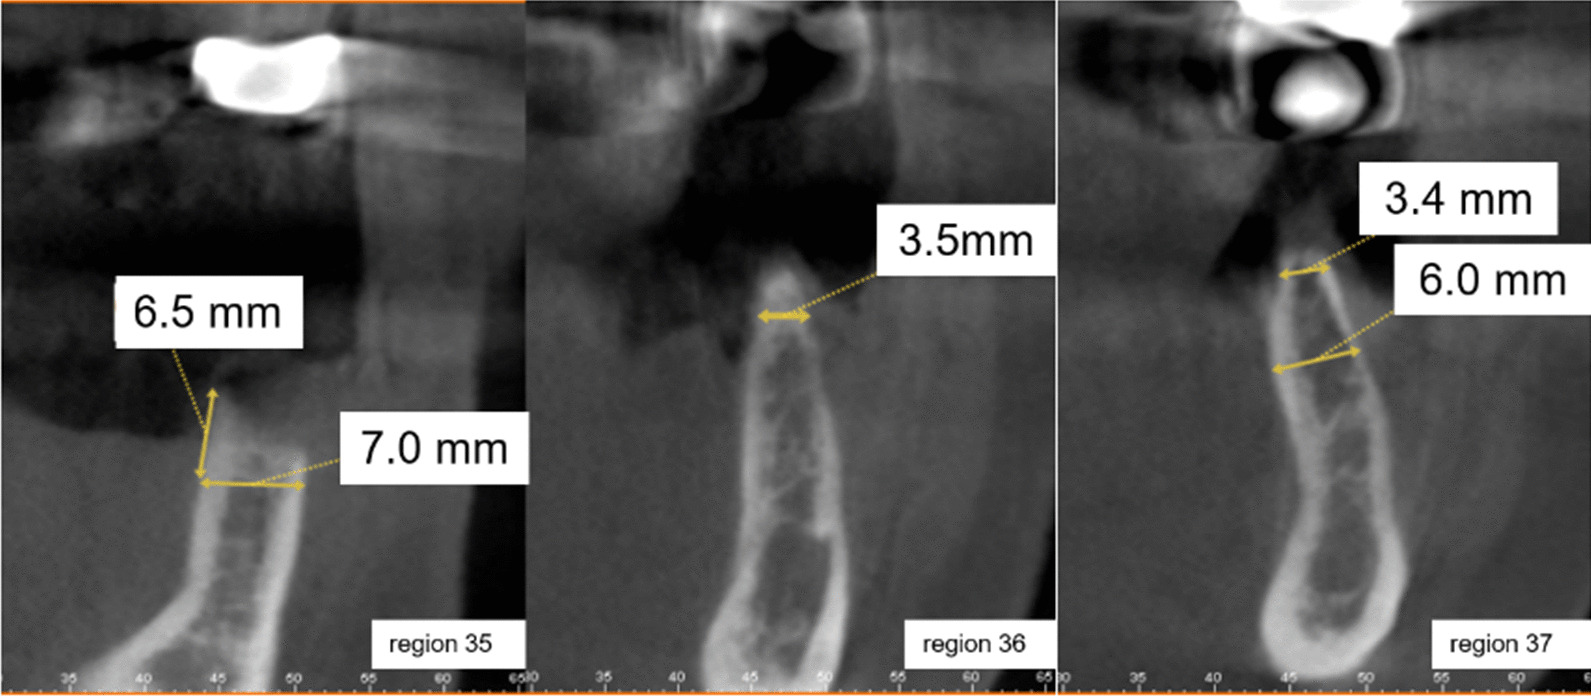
Cross-sections of regions 35–37 from the CBCT at 5 months after extraction of tooth 35. The alveolar ridge in region 35–37 is narrow in the crestal area and insufficient for implantation without pre-implantological treatment
